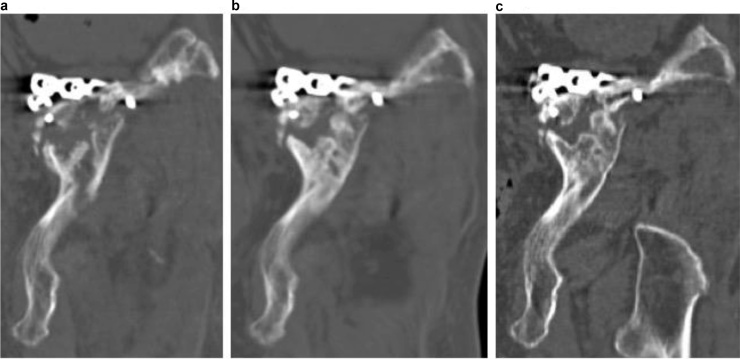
Post-operative computed tomography coronal views. Computed tomography images showing the process of union of the fracture site at (a) 5, (b) 12 and (c) 19 months after surgery reveal that union occurred between 12 and 19 months post-operatively.
